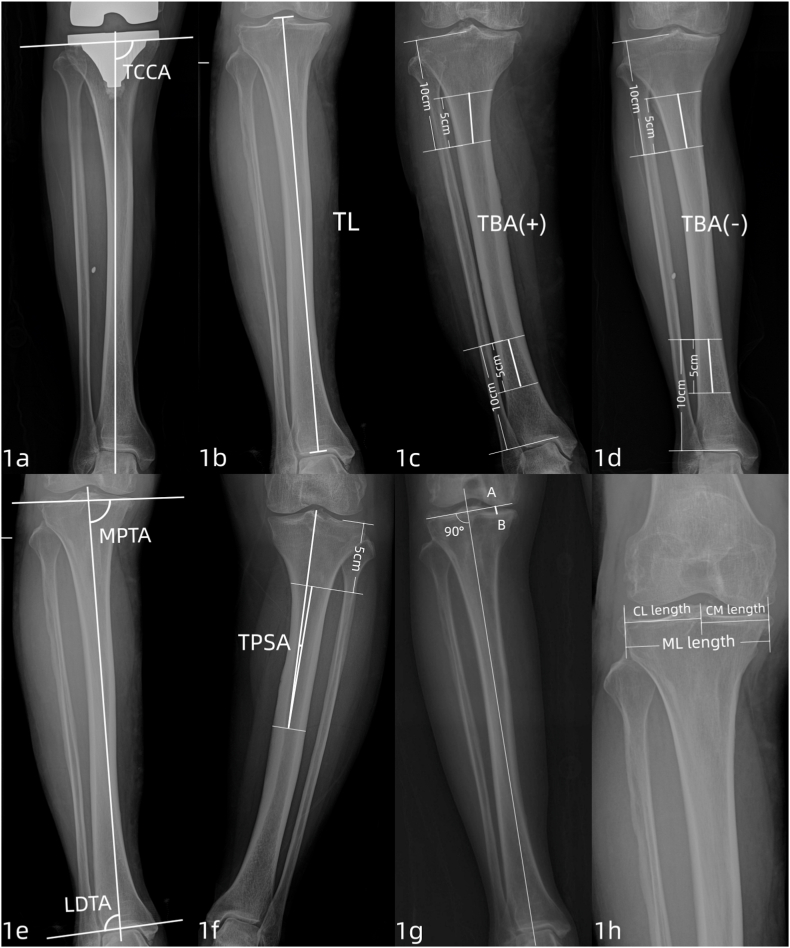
Imaging measurement is performed on a full-length hip-to-ankle radiograph. 1a TCCA, tibial component coronal alignment anglel; 1b Tibial length; 1c TBA, tibial bowing angle, the figure shows lateral bowing; 1d TBA, tibial bowing angle, the figure shows medial bowing; 1e MPTA, medial proximal tibial angle; LDTA, lateral distal tibial angle; 1f TPSA, tibial plateau shift angle; 1g tibial boss loss; 1h ML length, medial to lateral length; MC length, medial to center length; CL length, center to lateral length.
